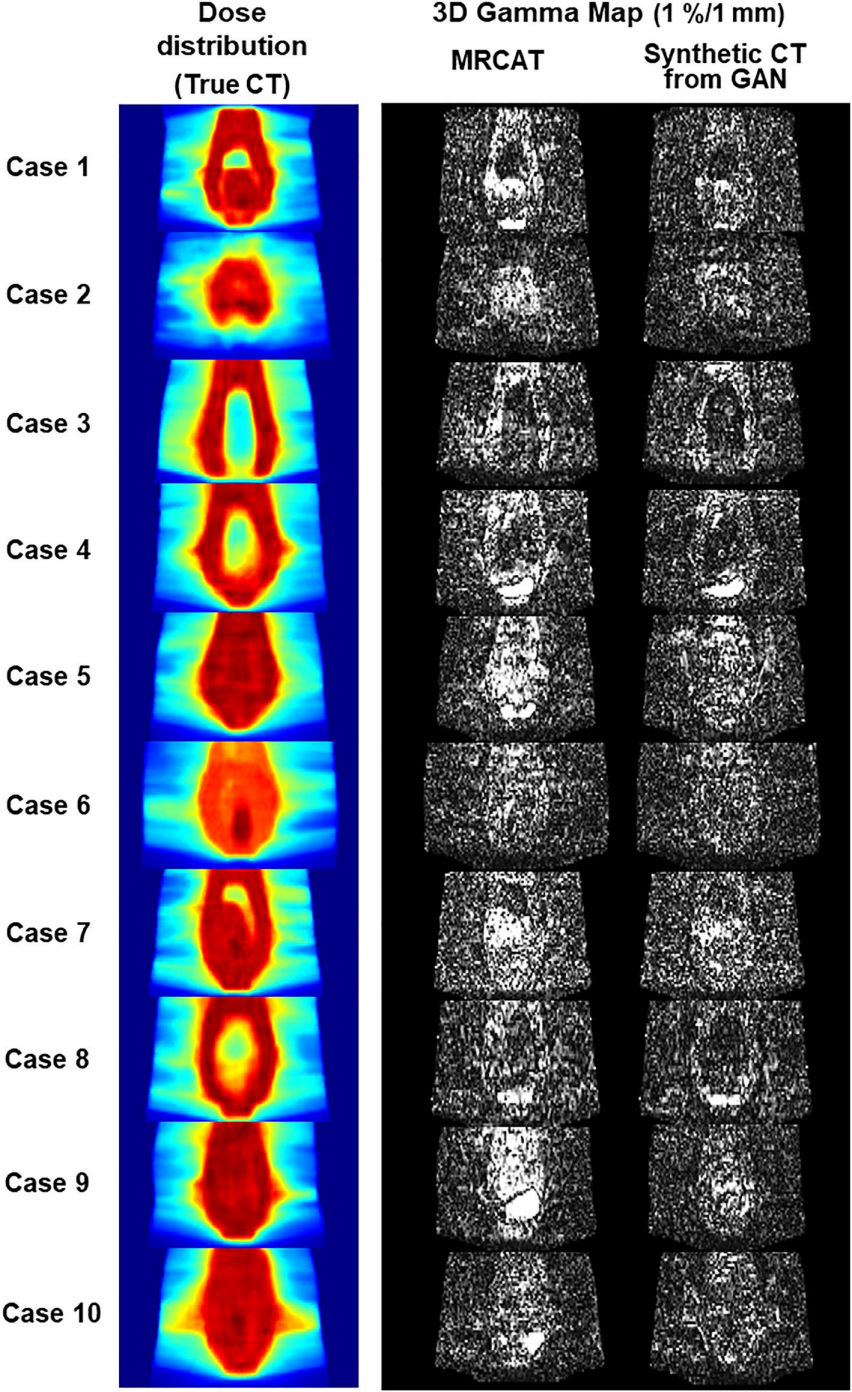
Dose distributions in a coronal plane on 10 testing cases (left column), and gamma maps (1%/1 mm criterion) between dose distributions on true CT and two types of synthetic CT images: MRCAT (middle column) and synthetic CT images from GAN (right column).
